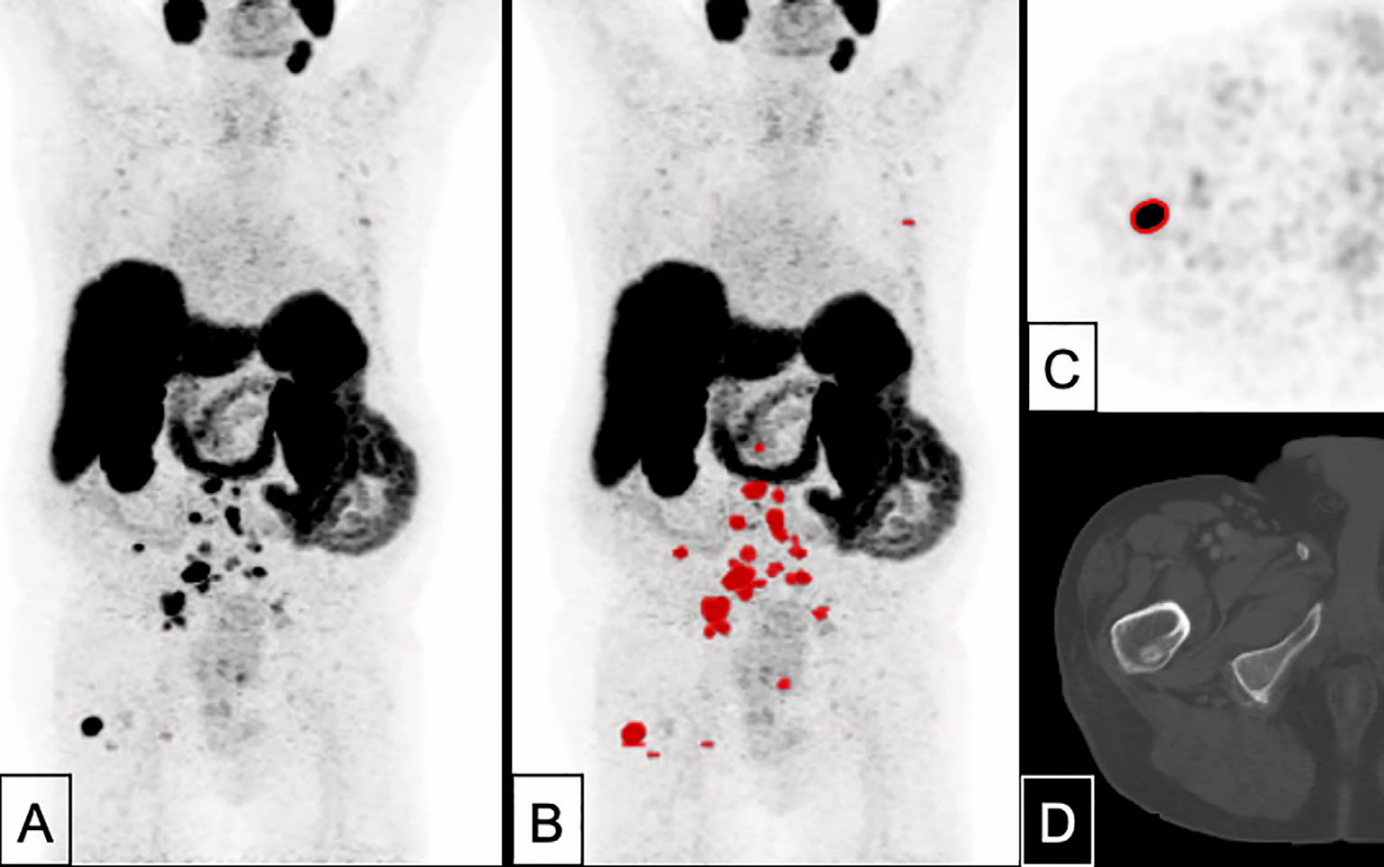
A 82 years-old patient with prostate cancer remnant as well as bone and lymph node metastases (PSA 10.1 ng/ml, Gleason 8). Tumor delineation using a cut-off of SUV 4.0 revealed a WTV of 37.9 ml. (A) maximum intensity projection (MIP); (B) MIP + WTV (red color); (C) delineation of a bone metastasis on PET; (D) CT correlate (bone window).
